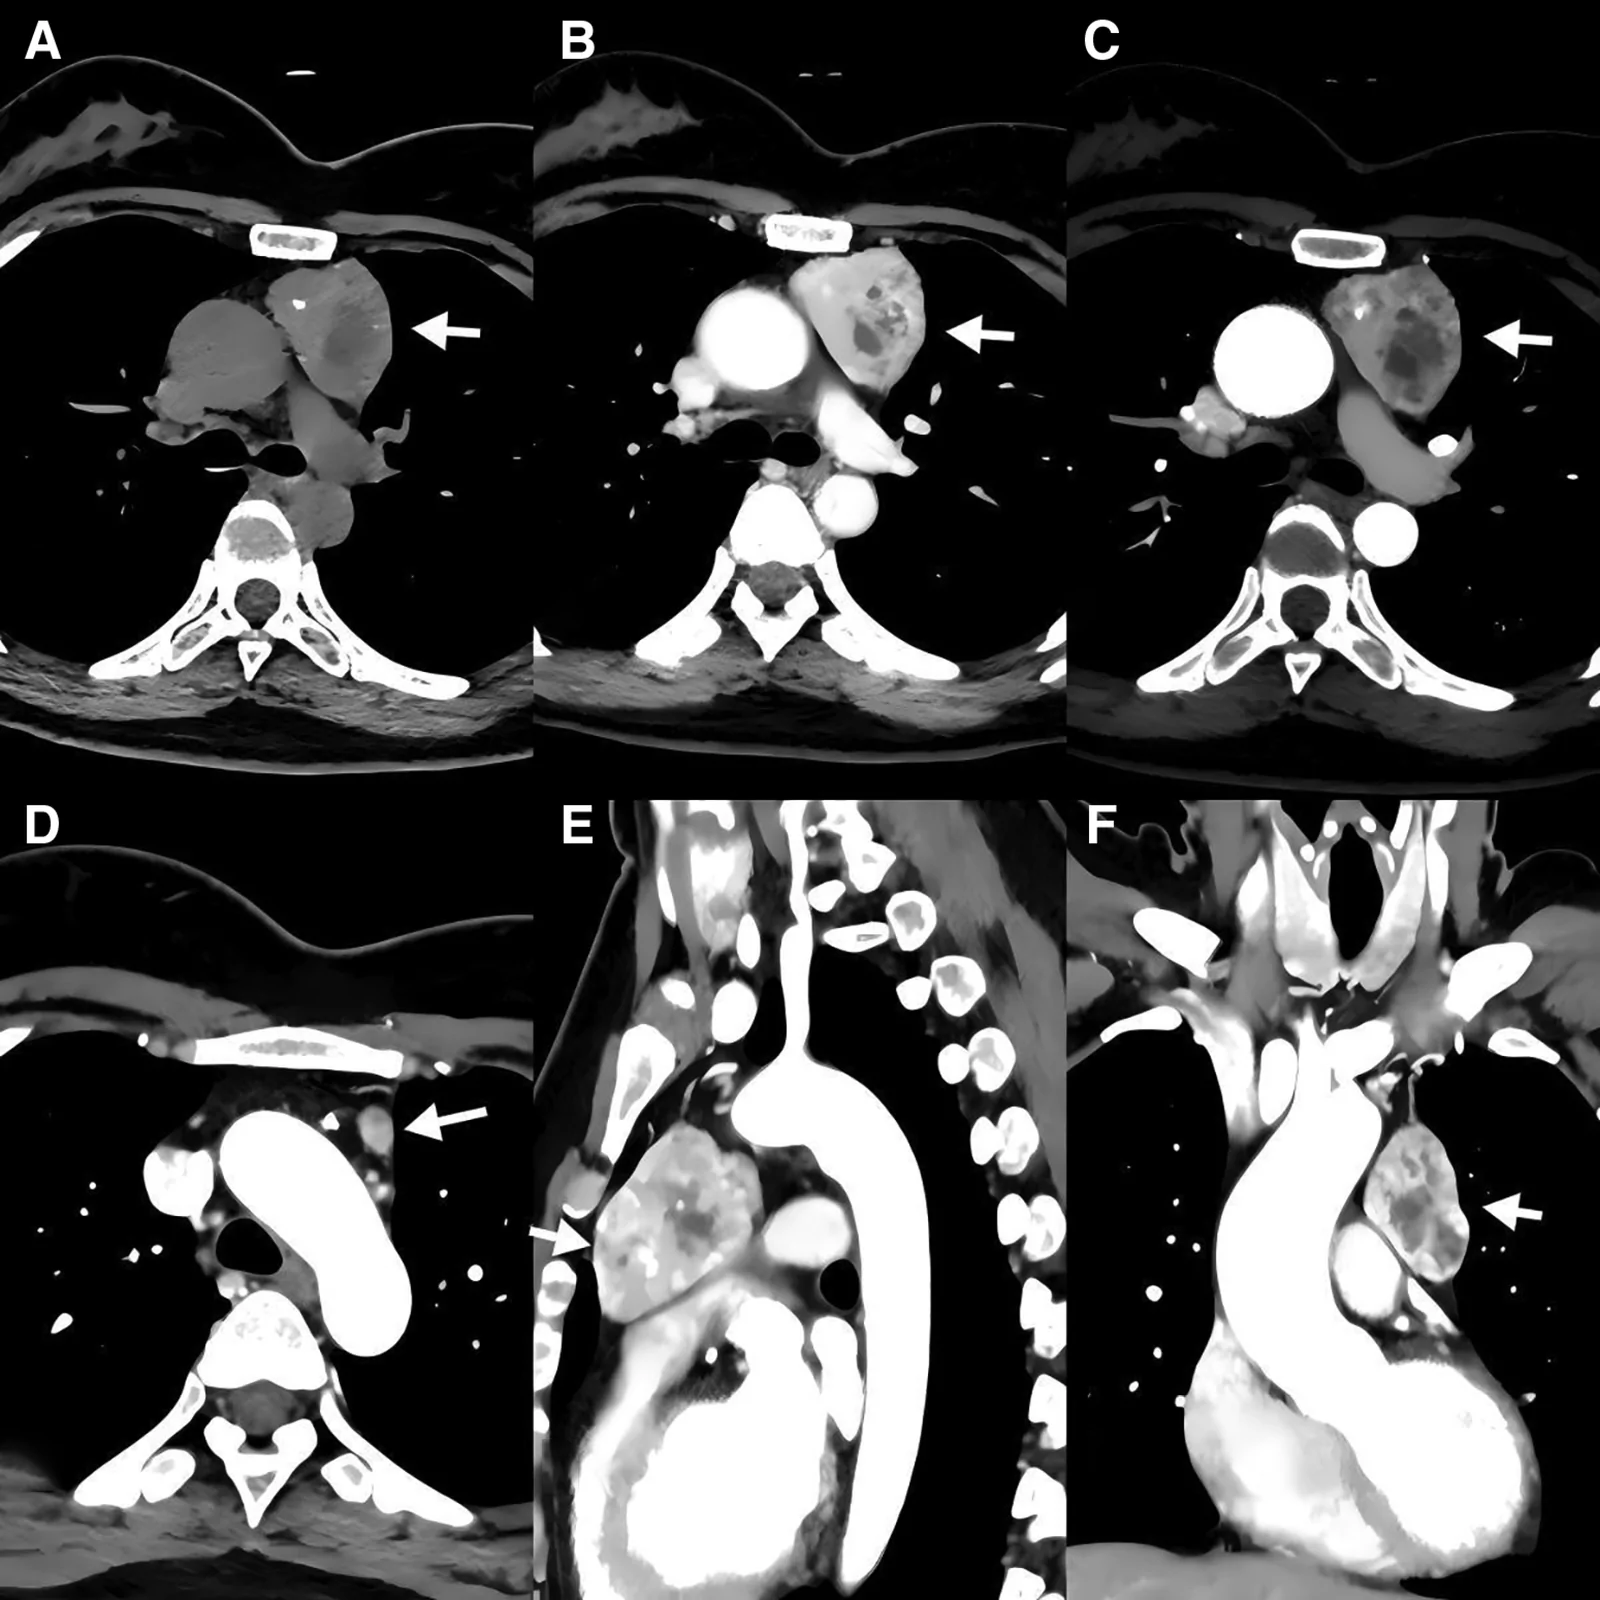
Chest CT and CTA findings of the anterosuperior mediastinal mass (white arrows). (A) Non-contrast axial CT: mean attenuation of the solid component 75.82 ± 18.46 HU. (B) Arterial-phase axial CT: marked, heterogeneous enhancement. (C) Venous-phase axial CT: partial washout. (D–F) Multiplanar reformations (axial, sagittal, coronal) and CTA demonstrate no anatomical continuity with the cervical thyroid and close adjacency to the left innominate vein; small-caliber vessels coursing along the superior aspect of the lesion are visualized on CTA (arrows). CT = computed tomography, CTA = computed tomography angiography, HU = Hounsfield unit.
